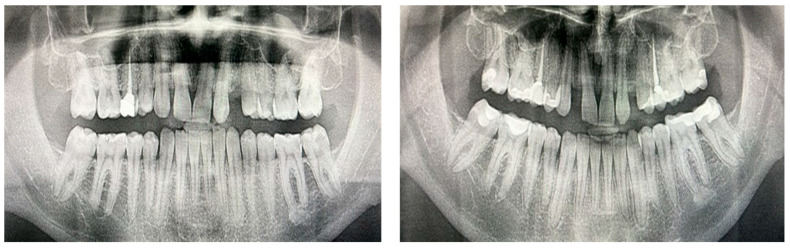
Representative panoramic radiographs of a patient before (left) and after clear aligner therapy (right). The treatment duration was 12 months (36 aligners). Slight apical resorption was visible on the maxillary incisors post-treatment, a common radiographic finding after orthodontic tooth movement, without clinical relevance.
